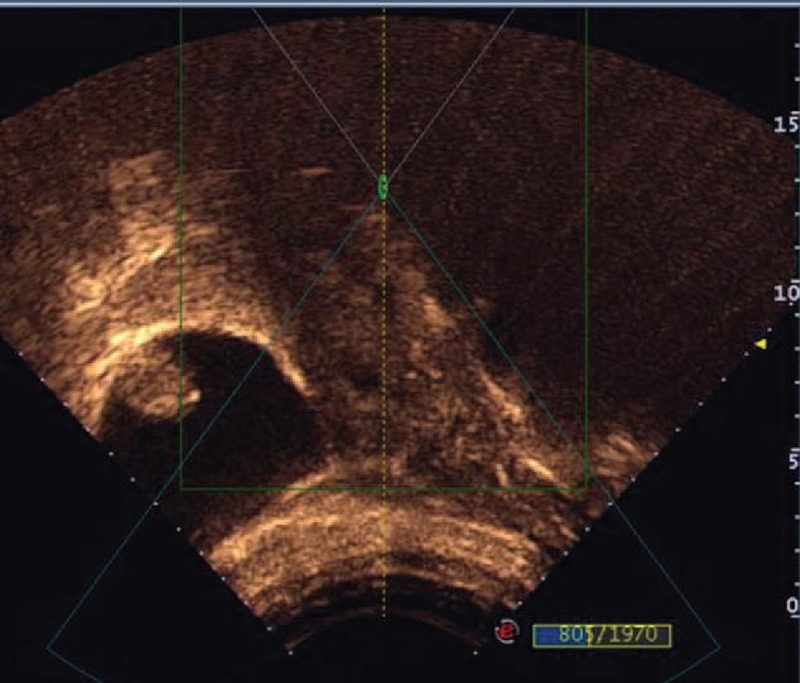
The blood flow of pregnancy tissue examined by transabdominal color Doppler scanning contrast medium revealed before high-intensity focused ultrasound ablation.
